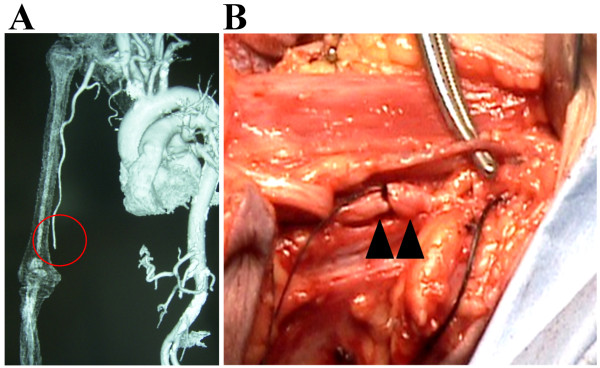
Above-elbow amputation 6 weeks after the emergency laparotomy. (A) The contrast-enhanced computed tomography. The brachial artery (circle) was occluded at the supracondylar level due to a thromboembolism. (B) An intraoperative photograph of the brachial artery. The arterial lumen is filled with an organized embolus.
